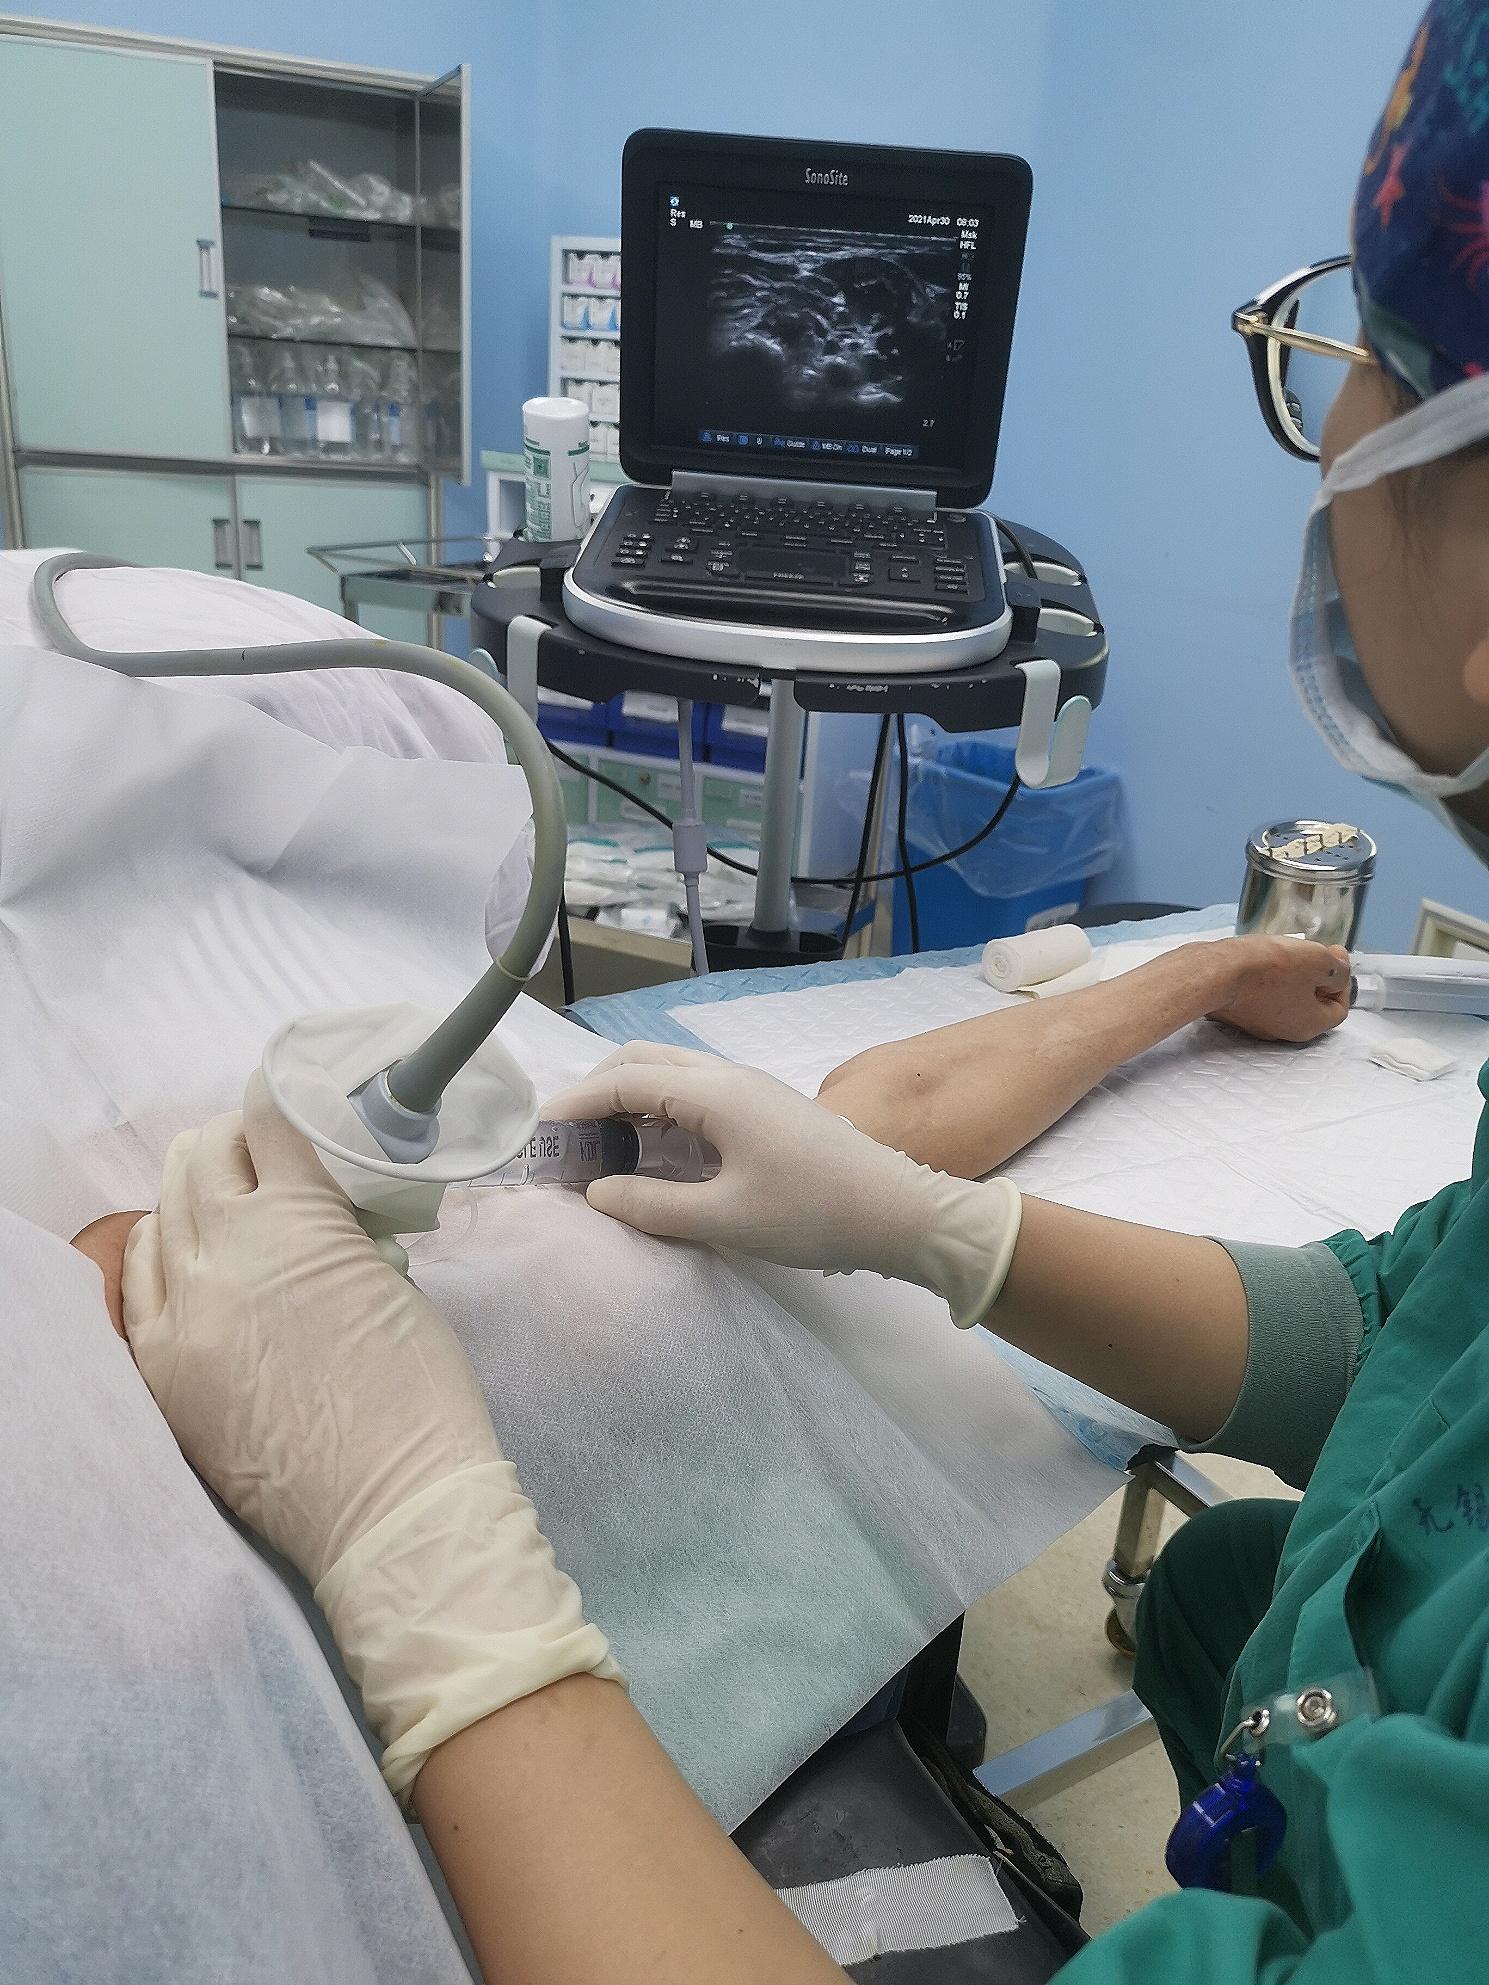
A cervical plexus block was administered under ultrasound guidance
